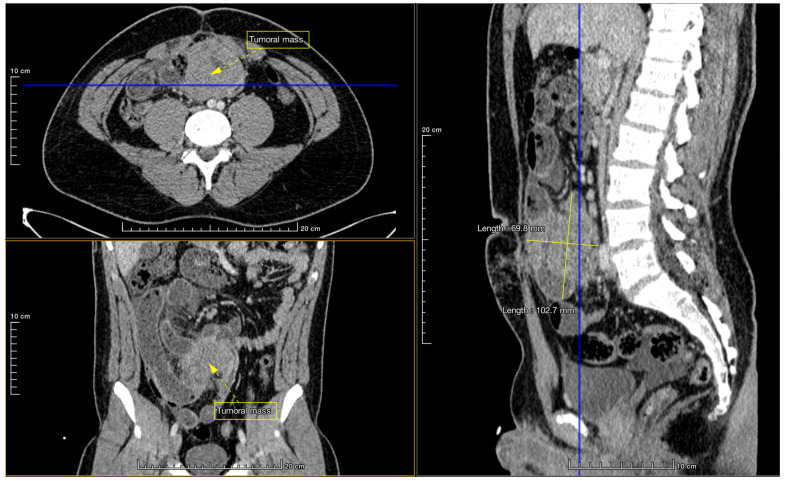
Preoperative CT scan: A loop of the ileum shows a thickened wall (tumoral mass) about 70 mm thick over a length of 10 cm, with findings associated with intravenous contrast media throughout the parietal volume. There is almost total stenosis of the lumen, possibly linked to nodular lymphoid hyperplasia, intestinal intussusception, or intestinal lymphoma, leading to intestinal occlusion. Multiple hydroaeric levels were observed in the small intestine, and enlarged mesenteric lymph nodes measuring up to 18 mm in diameter were present.
